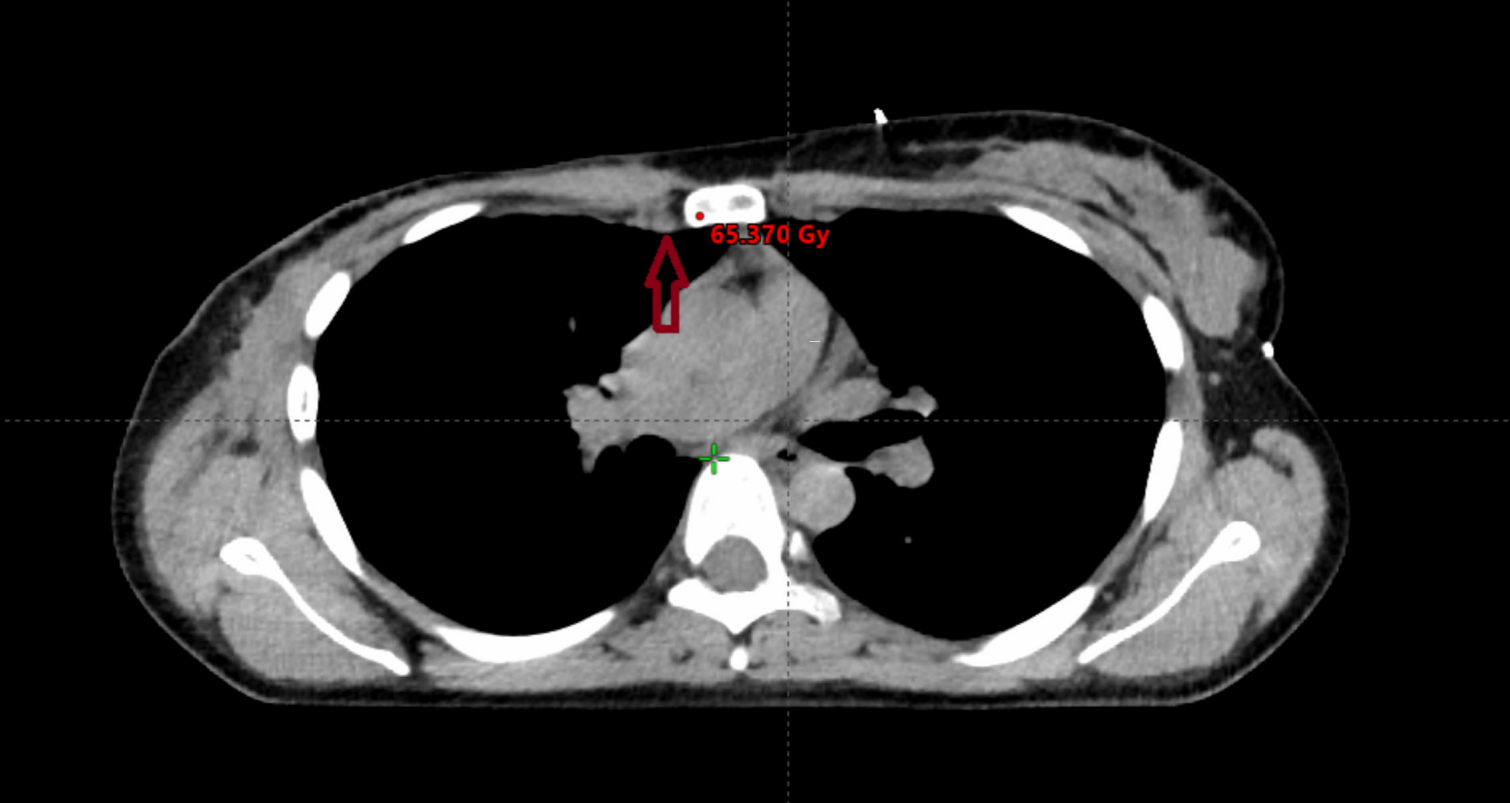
If primary systemic treatment (PST) is effective, remission will occur. On standard planning CT performed without intravenous contrast agent, the involved nodes may temporarily become almost invisible (dark red arrow). This image is from the same patient whose images are in the Figure 3 after systemic treatment and mastectomy.
